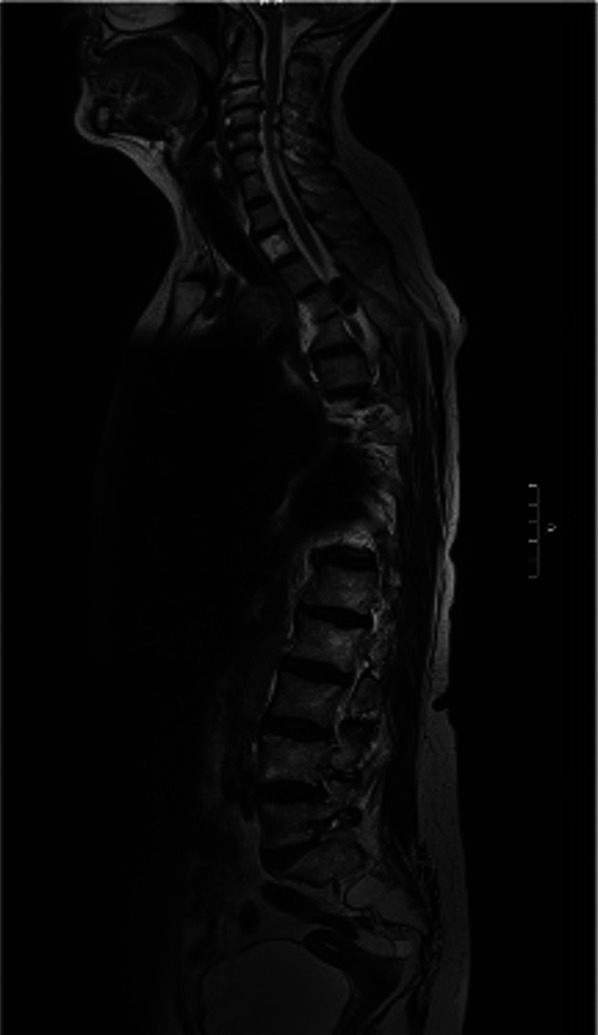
Left side sagittal slice of the whole spine T2-weighted MRI showing the neoplasm at the T4-T6 level in this patient with a T5 right hemivertebra.
